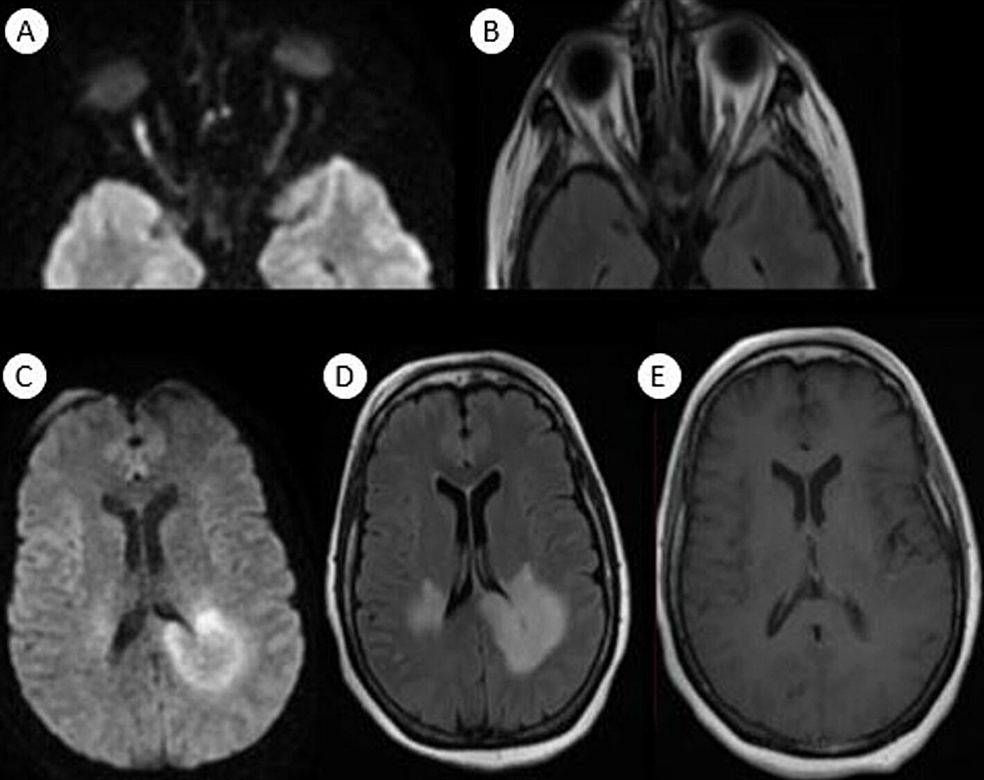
Orbital and cerebral magnetic resonance imaging after four weeks showed extension of previous lesions with involvement of the corpus callosum with diffusion restriction and peripheral enhancement, and appearance of bilateral optic neuritis more obvious on the right optic nerve than on the left one (A) Axial diffusion view of orbital magnetic resonance imaging; (B) axial T2 fluid-attenuated inversion recovery (FLAIR) view of orbital magnetic resonance imaging; (C) axial diffusion view of cerebral magnetic resonance imaging; (D) axial T2 FLAIR view of cerebral magnetic resonance imaging; (E) axial T1 Gado of cerebral magnetic resonance imaging.
